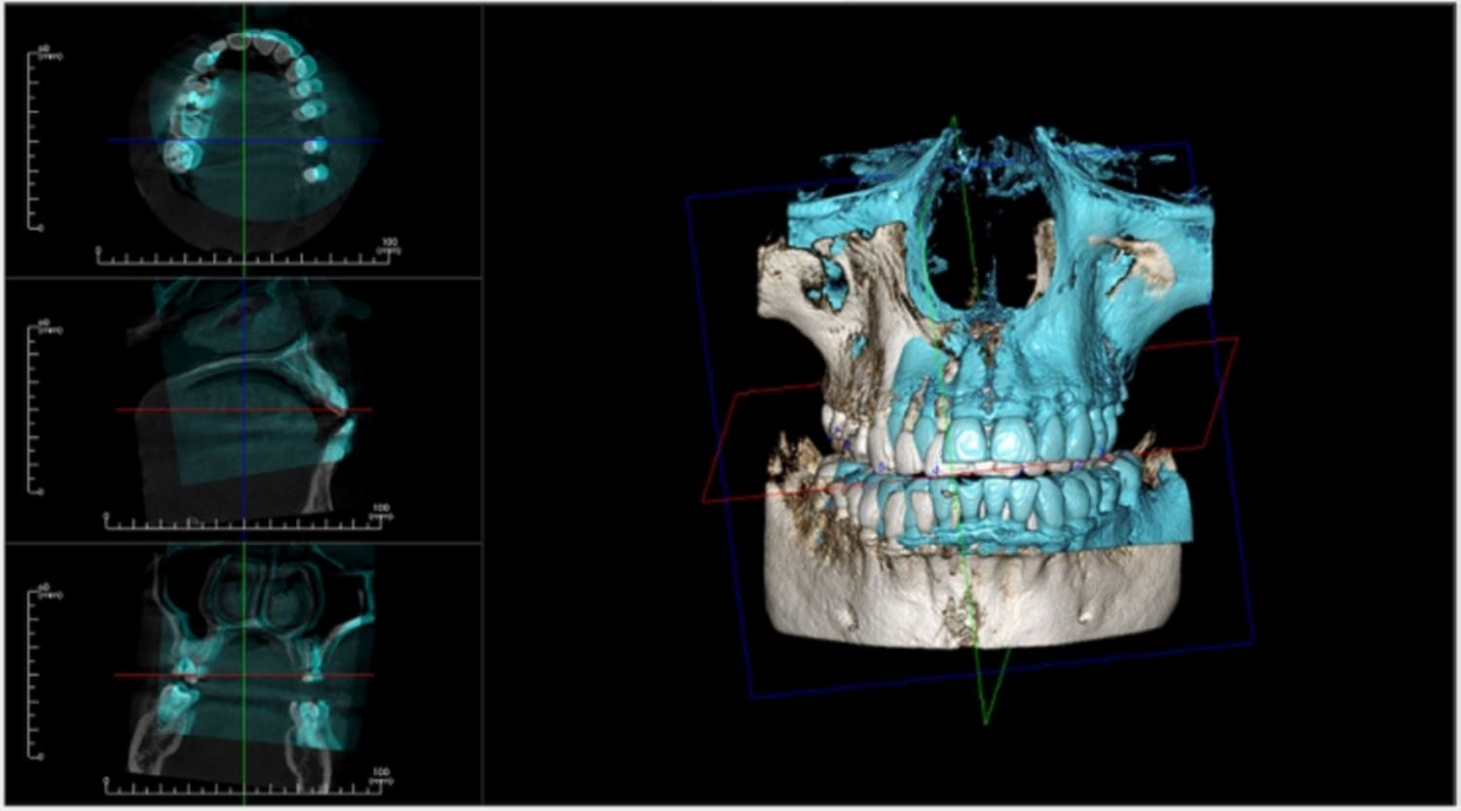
Showing the fusion of CBCTs
